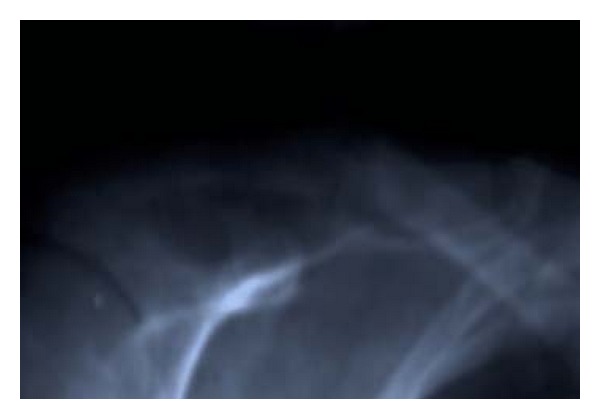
AP X-ray of the right clavicle showing osteitis fibrosa cystica lesion with a pathologic fracture (on admission).
